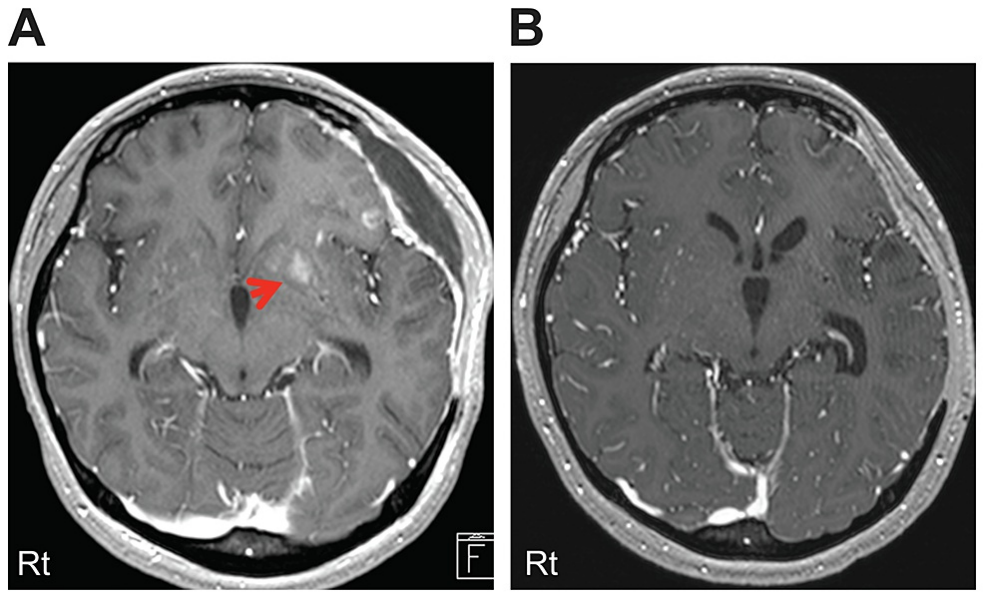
MRI findings (gadolinium enhancement) (A) Some gadolinium-enhanced lesions were observed in the frontal lobe before chemotherapy (arrow). (B) The gadolinium-enhanced lesions disappeared while the patient was on chemotherapy.
